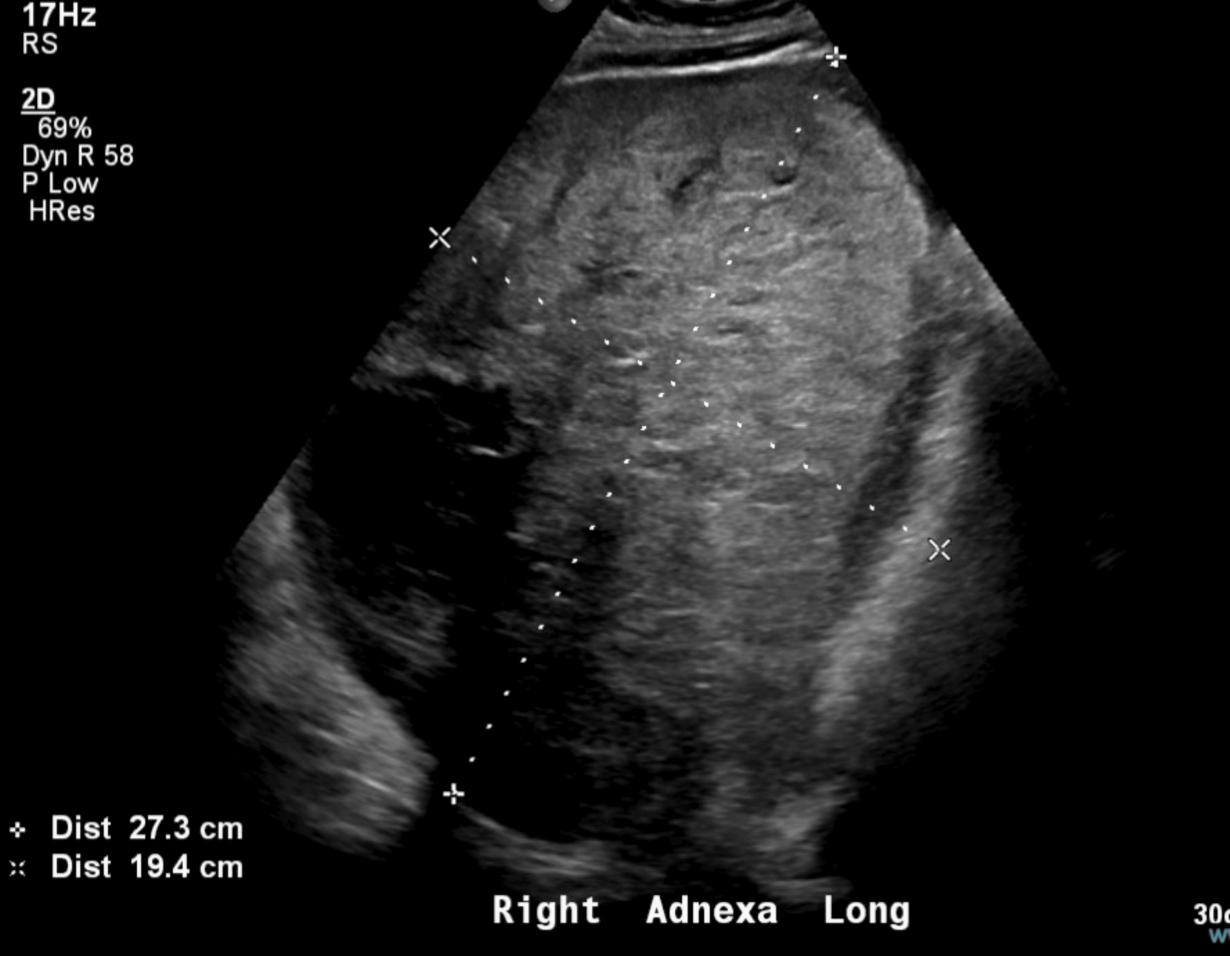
Transvaginal ultrasound of the right adnexa
